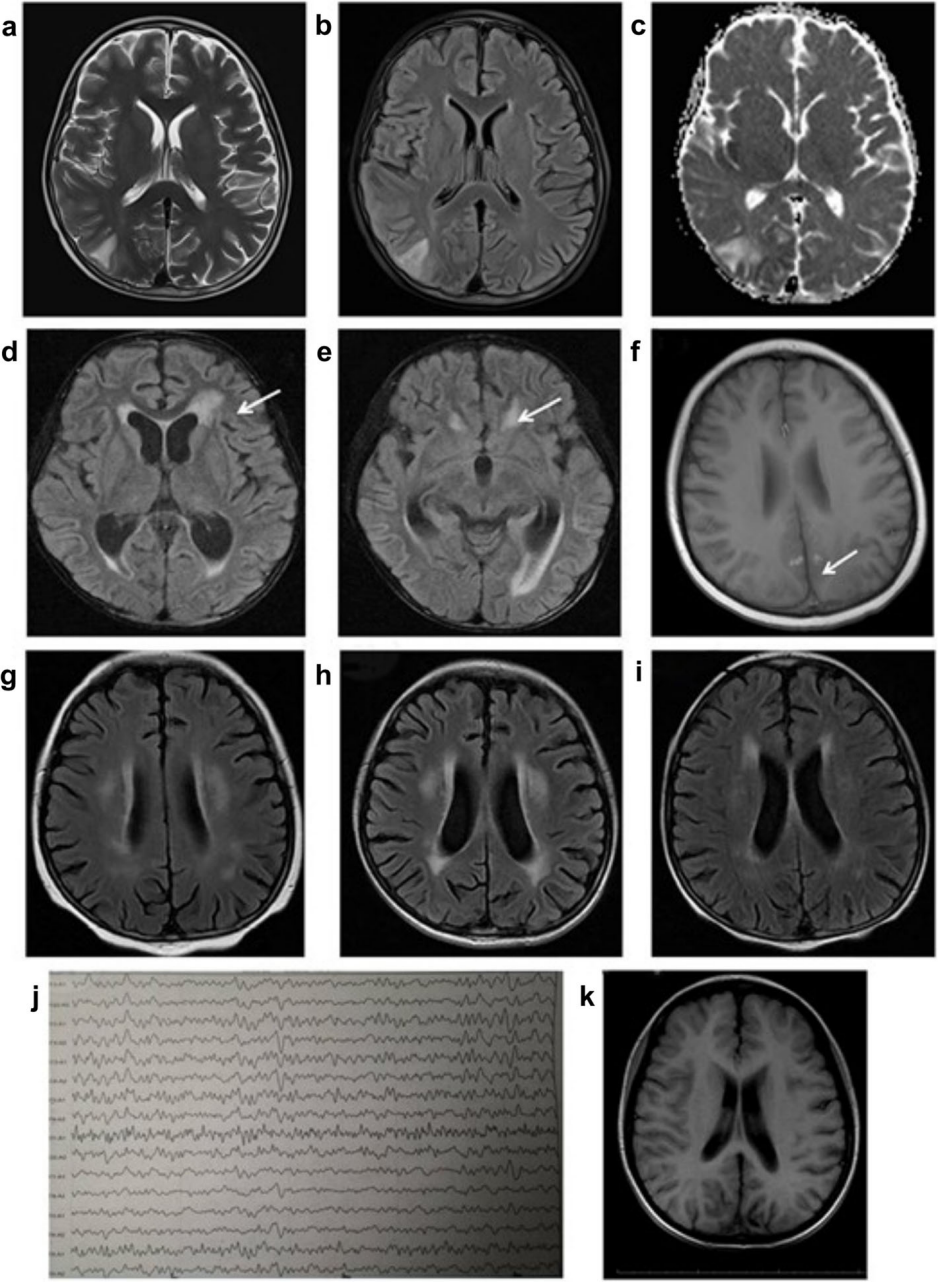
Brain magnetic resonance imaging (MRI) and electroencephalography. a, b, c MRI of the brain of patient #1 showing asymmetrical involvement of the cortex and subcortical white matter with high-intensity signals evident on axial T2-weighted. a T2-fluid attenuation inversion recovery (T2-FLAIR). b Apparent diffusion coefficient (ADC). c Scans. Similar MRI findings were obtained in five other patients with cyclosporine A-related neurotoxicity (patients #2, #3, #4, #5 and #7). d and e. Axial FLAIR MRI of the brain of patient #6 (obtained 3 days after the onset of symptoms) showing hyperintense signals in the periventricular white matter (white arrow in d and e) as well as the thalamus (blue arrow in d) and hippocampus (blue arrow in e). f Axial T1-weighted MRI of the brain of patient #4 obtained 3 days after the onset of symptoms. This patient had cerebral hemorrhage associated with posterior reversible encephalopathy syndrome that manifested as a parenchymal hematoma and small hemorrhages < 5 mm in size. The white arrow indicates a microbleed in the occipital lobe. g, h and i. Follow-up MRI of the brain of patient #2. g Patchy shadows with high signal intensity were observed in the lateral periventricular white matter 3 days after the onset of symptoms. h Hyperintense patchy shadowing in the lateral periventricular white matter was increased at 1 month after onset, despite an improvement in clinical symptoms at this time. i Patchy shadowing was less evident at 6 months after symptom onset. j and k. Electroencephalography and MRI findings in patient #3. MRI showed local cortical atrophy in the left parietal and occipital lobes, and the electroencephalogram demonstrated a spike rhythm originating from the same region of the brain
